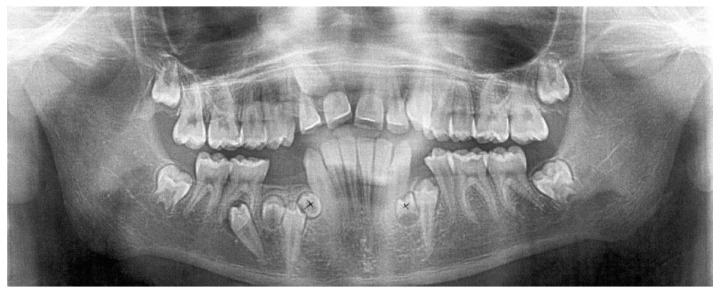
Panoramic radiograph, taken one year later, when patient was 12 years old, showing three additional mandibular premolars: the first one located between the apex of the tooth 33 and the crown of the impacted tooth 34; the second one, found between the apex of the root of the tooth 43 and the crown of the impacted tooth 44; and the third one, found between the mesial root of the tooth 46 and the crown of the impacted tooth 44. The distal migration of the tooth 45 was noticeable and its crown was located in the projection of the roots of the tooth 46. The tooth 13 was located between 1/3 of the cervical part of the root of the tooth 12 and the tooth 11. Alpha and beta angles of the tooth 13 increased to 29° and 20°.
